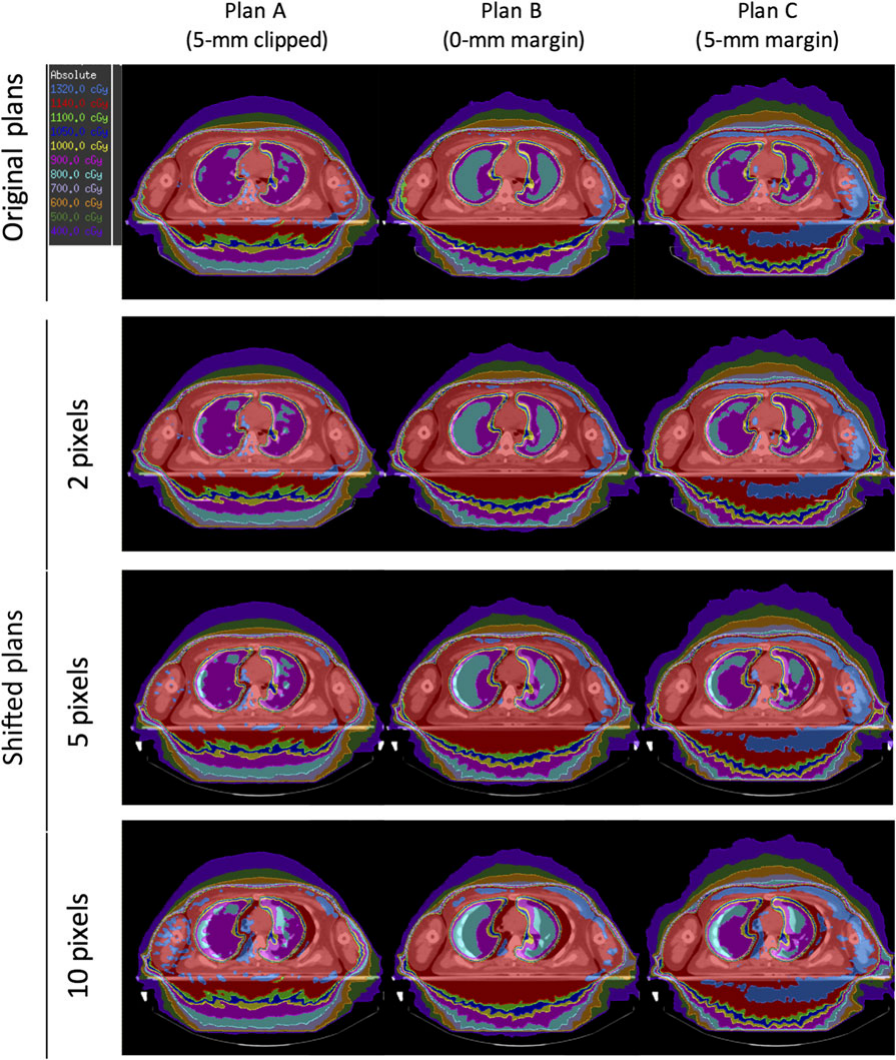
Examples of dose distributions. In Plan C, the area just under the skin surface tends to receive a high irradiation dose. When CT images are shifted to the left side, the body is exposed to a high-fluence beam and the high-dose area is enlarged.
